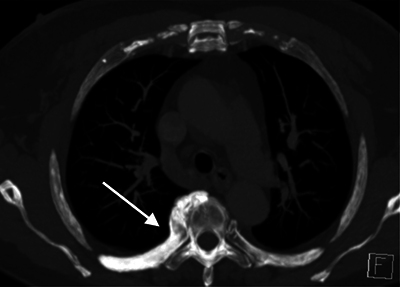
CT thorax axial MIP images.
